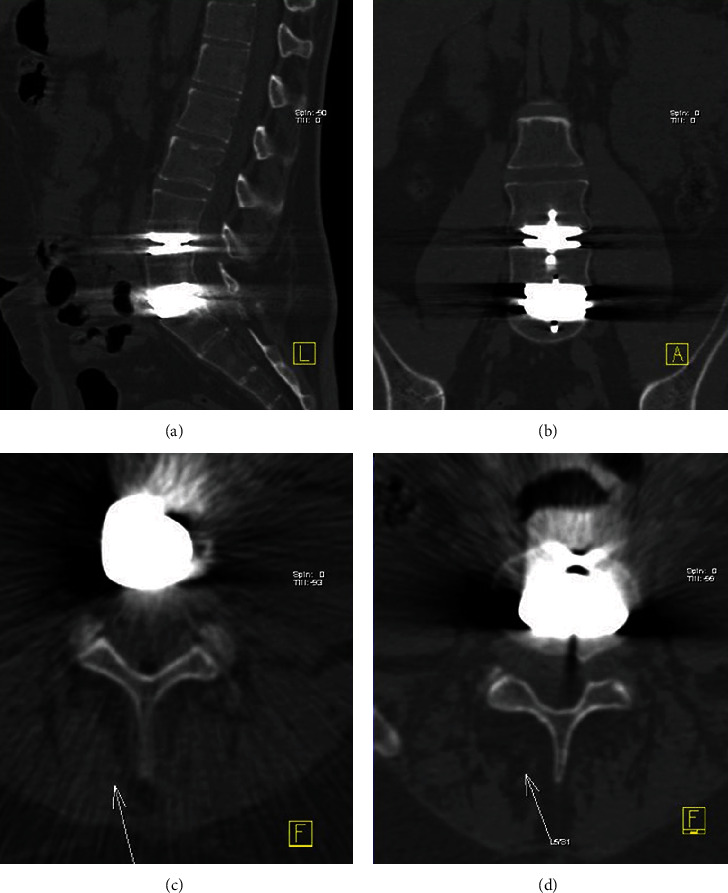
Examples of CT beam hardening artifacts caused by a metallic prosthesis in the lumbosacral (a, b) and cervical (c, d) spine.
